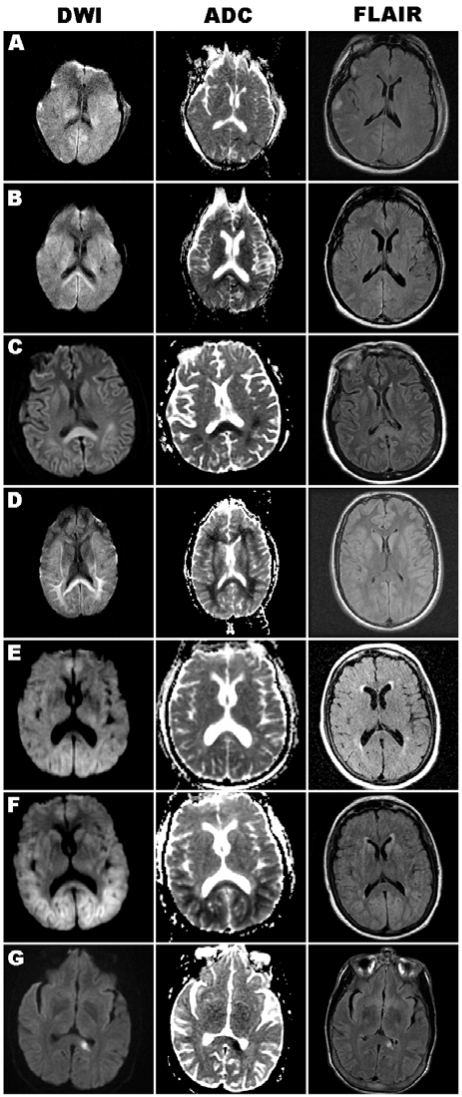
Axial brain MRI images of DWI, ADC, and FLAIR sequences obtained in each case. A, Case 1, early MRI (Day 3) without splenium or cortical diffusion signal. B, Repeat MRI of case 1 (Day 10), with bilateral DWI-bright and ADC-dark splenium signal. C, Case 2 (Day 7), prominent DWI-bright and ADC-dark splenium signal, with subtle cortical ribbon signal, hyperintensity of these regions also present on FLAIR sequence. D, Case 3 (Day 8), DWI-bright and ADC-dark signal in the splenium and subcortical white matter, abnormal signal not well seen on FLAIR. E, Case 4, early MRI (Day 1) with minimal pathology. F, Case 4, late MRI (Day 6) with DWI-bright and ADC-dark signal in the splenium and occipital/parietal regions, with subtle corresponding hyperintensity signal on FLAIR. G, Case 5 (Day 3), isolated ischemic lesion in the left splenium, seen on all three sequences.
